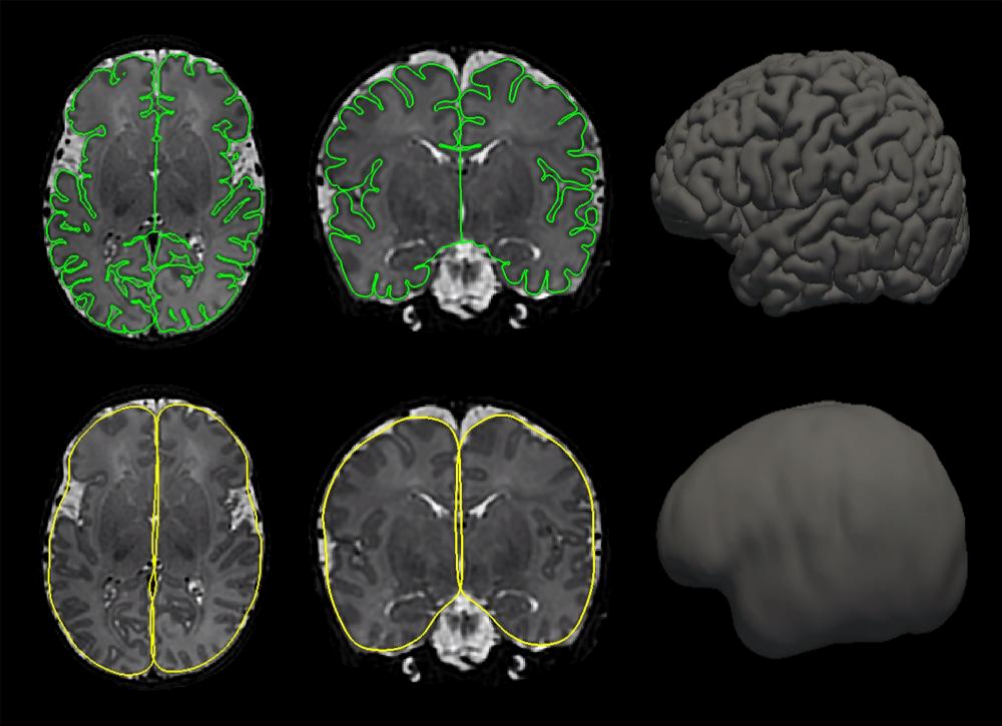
Cortical surfaces. Examples of the left and right hemisphere pial surfaces (green) and outer smoothed surfaces (yellow) used for calculation of the GI, overlaid on T2-weighted brain MRIs for one subject, acquired at 39^+3^ weeks. The surface renderings of both the pial and outer smoothed surfaces are shown alongside.
